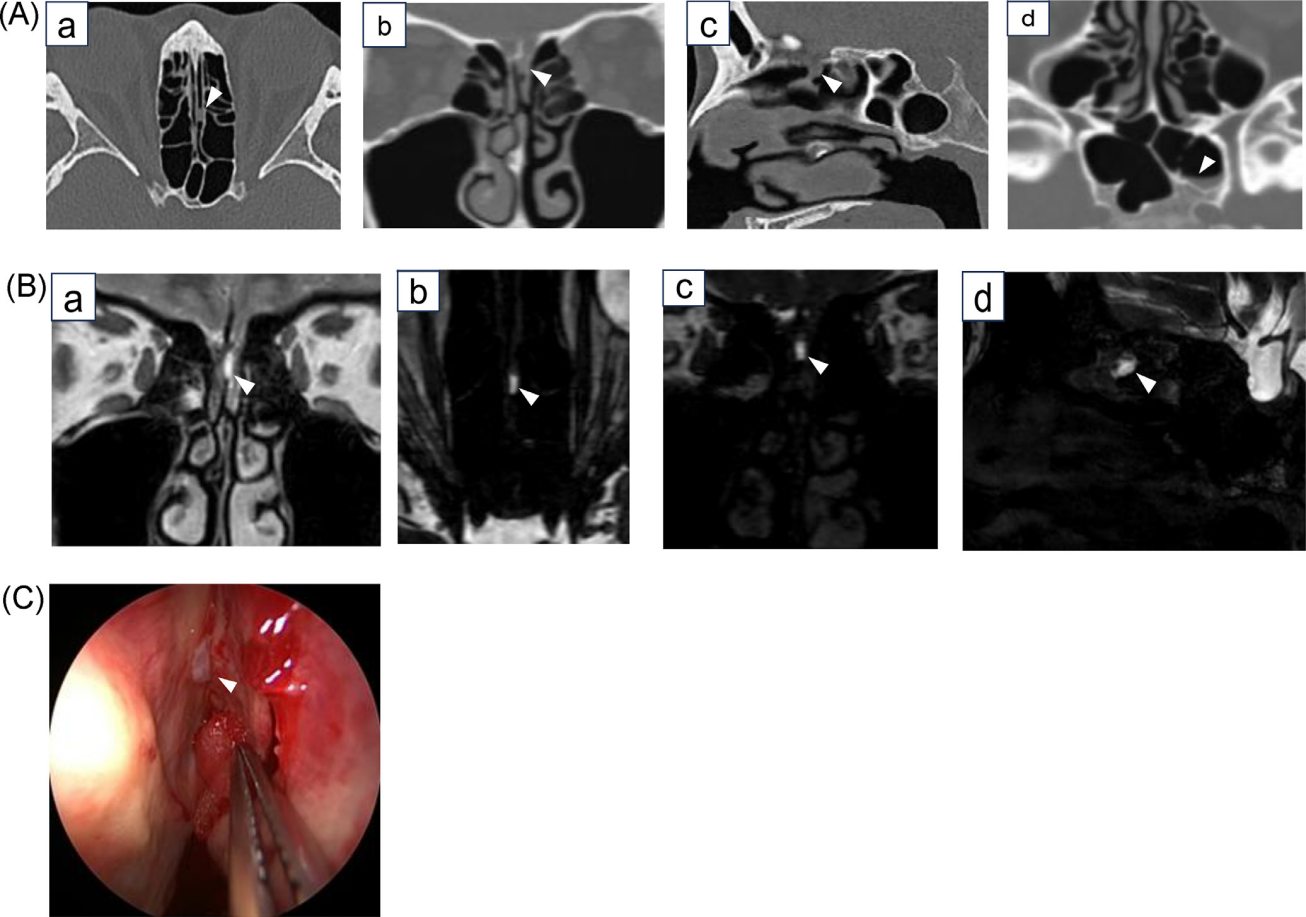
CT. (a) ) Oblique axial plane aligned parallel to the base of the skull. (b) Oblique coronal plane aligned perpendicular to the base of the skull. (c) Oblique sagittal plane aligned with the left olfactory fissure. (d) Axial plane. The left cribriform plate was unclear, and there was a soft tissue density continuous with the subarachnoid space (a-c) (arrowheads). An accumulation in the left sphenoid sinus was thought to be leaked CSF (d) (arrowhead). 2B. MRI. (a) Coronal T2WI. (b) 3D-MR cisternography oblique axial plane aligned parallel to the base of the skull. (c) 3D-MR cisternography oblique coronal plane aligned perpendicular to the base of the skull. (d) 3D-MR cisternography oblique sagittal plane aligned with the left olfactory fissure. T2WI and 3D-MR cisternography showed high signal intensity in the left olfactory fissure, suggesting fluid (a-d) (arrowheads). However, communication with the subarachnoid space was not evident. 2C. Endoscopy. A meningocele (arrowhead) between the left superior nasal turbinate and the nasal septum was observed. The fistula measured approximately 1-2 mm in size. CT, computed tomography; CSF, cerebrospinal fluid; MRI, magnetic resonance imaging; T2WI, T2-weighted imaging; 3D, three-dimensional.
